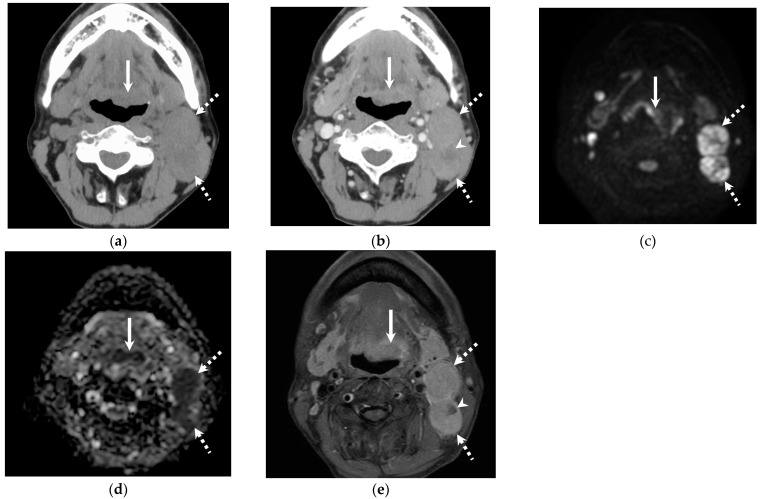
Representative images of a 58-year-old male with HPV-negative OPSCC in the base of the tongue. Local recurrence and pulmonary metastasis occurred. (a) Unenhanced CT shows mass in the base of the tongue (primary lesion, arrow) and multiple left upper jugular lymph node metastases (dotted arrow). CT attenuation of the primary lesion shows +44 HU. (b) Contrast-enhanced CT shows primary lesion and nodal metastasis. CT attenuation of nodal metastasis demonstrated + 75 HU. (c,d) Diffusion-weighted imaging and ADC map show high signal intensity and low ADC value. ADC value of the nodal metastasis was 0.715 × 10^−3^mm^2^/s. (e) Fat-suppressed contrast-enhanced T1-weighted image shows primary lesion (arrow) and multiple nodal metastases (dotted arrow) with necrosis (arrowhead). The SIR of solid component of the lymph node is 1.80.
